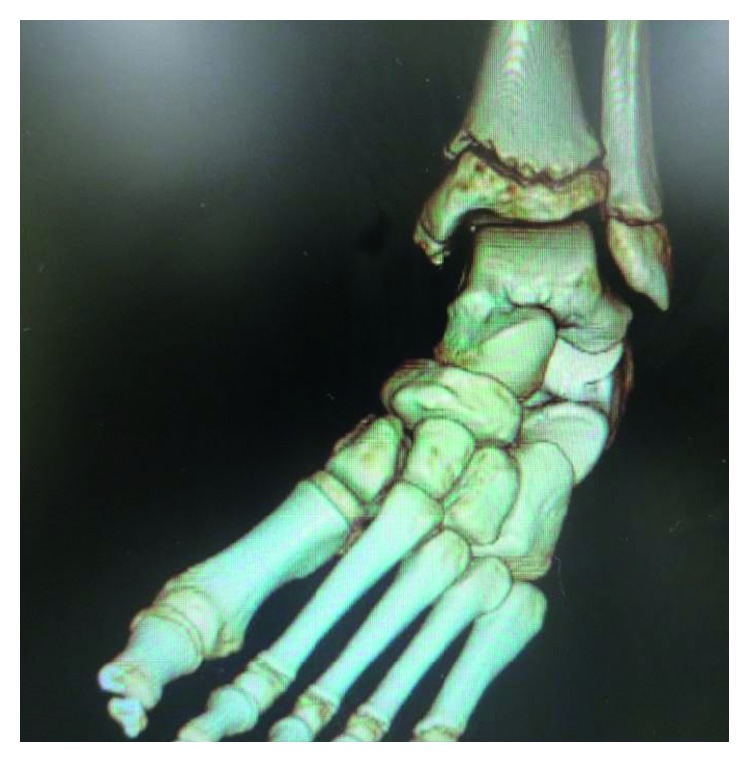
Dislocation of the left Chopart joint complex can be seen on the frontal computed tomography image.
